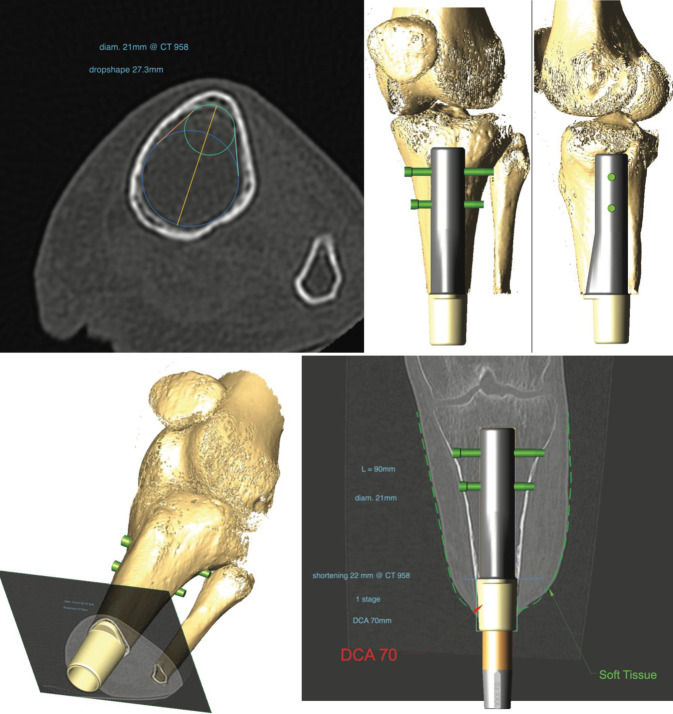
Preoperative surgical planning based on computed tomography (CT) scan images to configure the template tibial remnant
